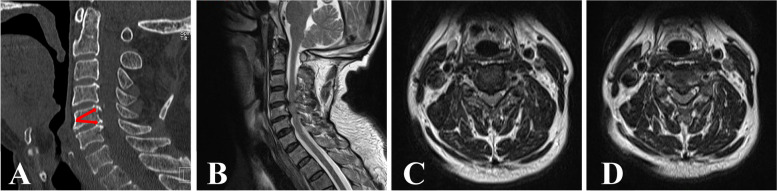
A 48-year-old male was examined for adjacent two-segment CSM. Preoperative sagittal CT image showed severe osteophytes posterior to C4-5 and C5-6 (A). The two red arrows in the figure indicate the trajectory of the intended bone channel. Preoperative MRI sagittal images showed that the disc-osteophyte complex in C4-5 and C5-6 significantly compressed the spinal cord (B). Preoperative MRI axial image of C4-5 (C) and preoperative MRI axial image of C5-6 (D)
